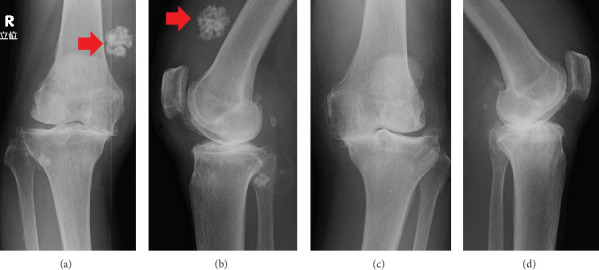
Preoperative plain radiograph with osteoarthritis of the bilateral knees. (a, b) Right knee and (c, d) left knee. (a, c) Anteroposterior view and (b, d) lateral view of the bilateral knees. Synovial osteochondromatosis was confirmed on the right knee (red arrow).
